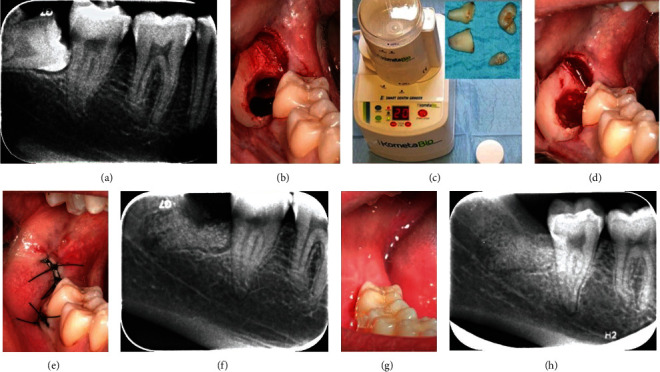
Presurgical periapical radiograph of the right third molar (test site) (a). Fresh post-extractive socket (b). The Smart Dentin Grinder™ (c) used for the test and the fragments of the tooth before being grinded (square). The dentin particulate filling the socket (d). The sutured wound (e). Periapical radiograph of the grafted socket taken after 15 days from the surgery (f). Soft (g) and hard (h) tissues healing at 180 days after surgery.
